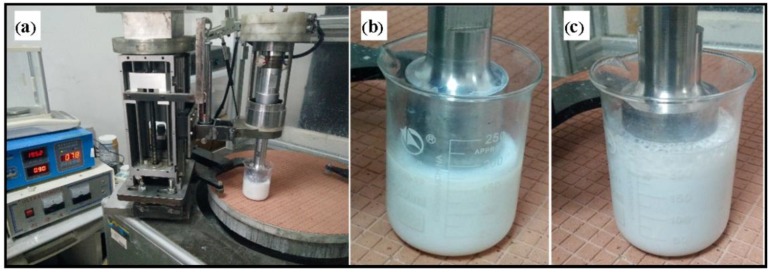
Physical picture of chemical corroding using CMP slurry: (a) Equipment for chemical corroding; (b) conventional chemical corroding; (c) ultrasonic chemical corroding.
